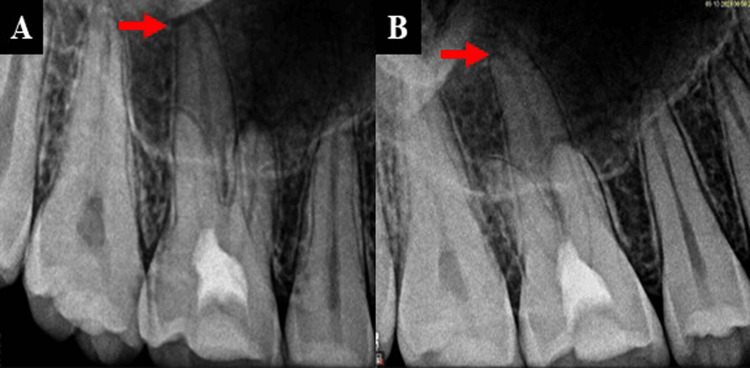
(A) Three-month follow-up radiograph and (B) six-month follow-up radiograph.
